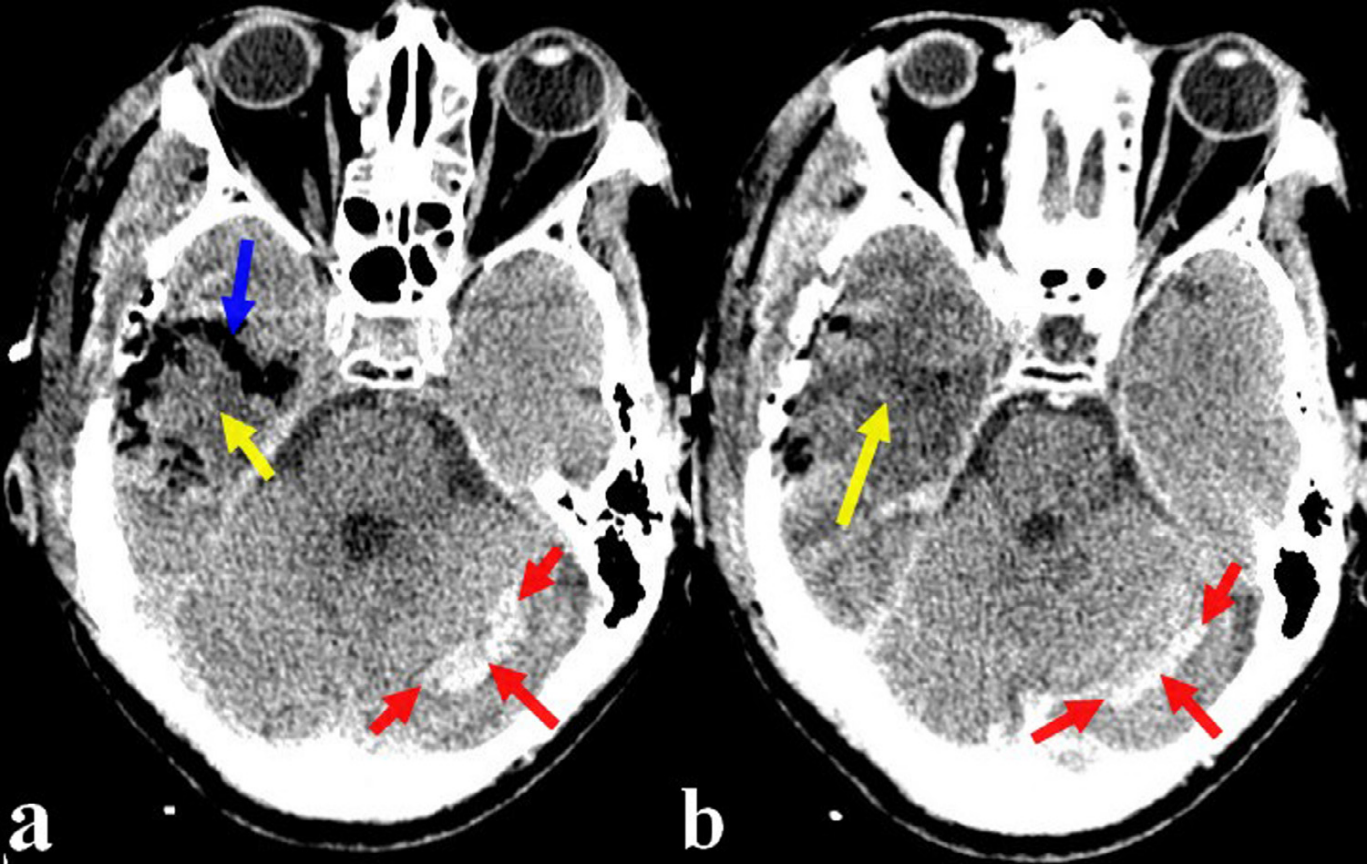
Brain CT scan in axial plane and parenchymal window before (a) and after enhancement (b) performed 24 hours postoperatively showing a postoperative oedemato-hemorrhagic change (a, b; yellow arrows) without obvious radiological signs of residual tumor associated with right temporal pneumocephalus (a; blue arrow). The whole was associated with a left cerebellar hematoma of 11 × 13 mm in diameter (a, b; red arrows) without a mass effect on the fourth ventricle.
